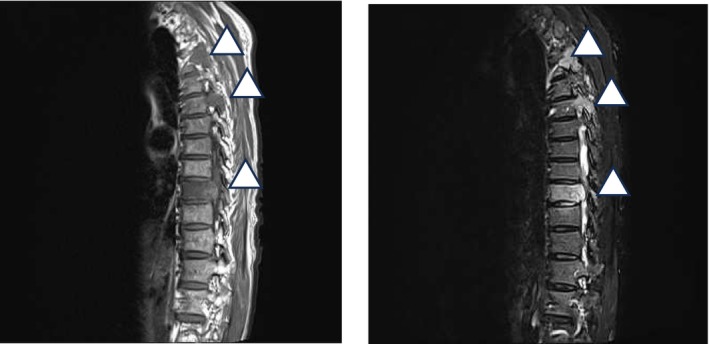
Magnetic resonance imaging reveals metastatic bone tumors at the levels of Th3, Th5, and Th9, compressing the spinal cord.
